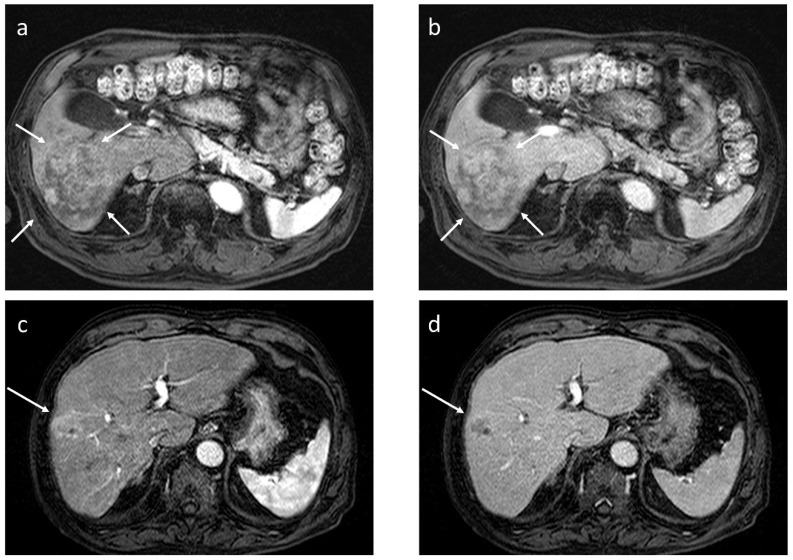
Dynamic gadoxetate-enhanced MRI in two representative cases ((a,b) and (c,d)). Both lesions exhibit arterial phase hyperenhancement (a,c), but in the former, there is no portal phase washout ((b) arrows), whereas in the latter, there is apparent portal phase washout ((d) arrow); the former pathologically turned out to be well to moderately differentiated hepatocellular carcinoma (HCC), and the latter to be poorly differentiated HCC.
